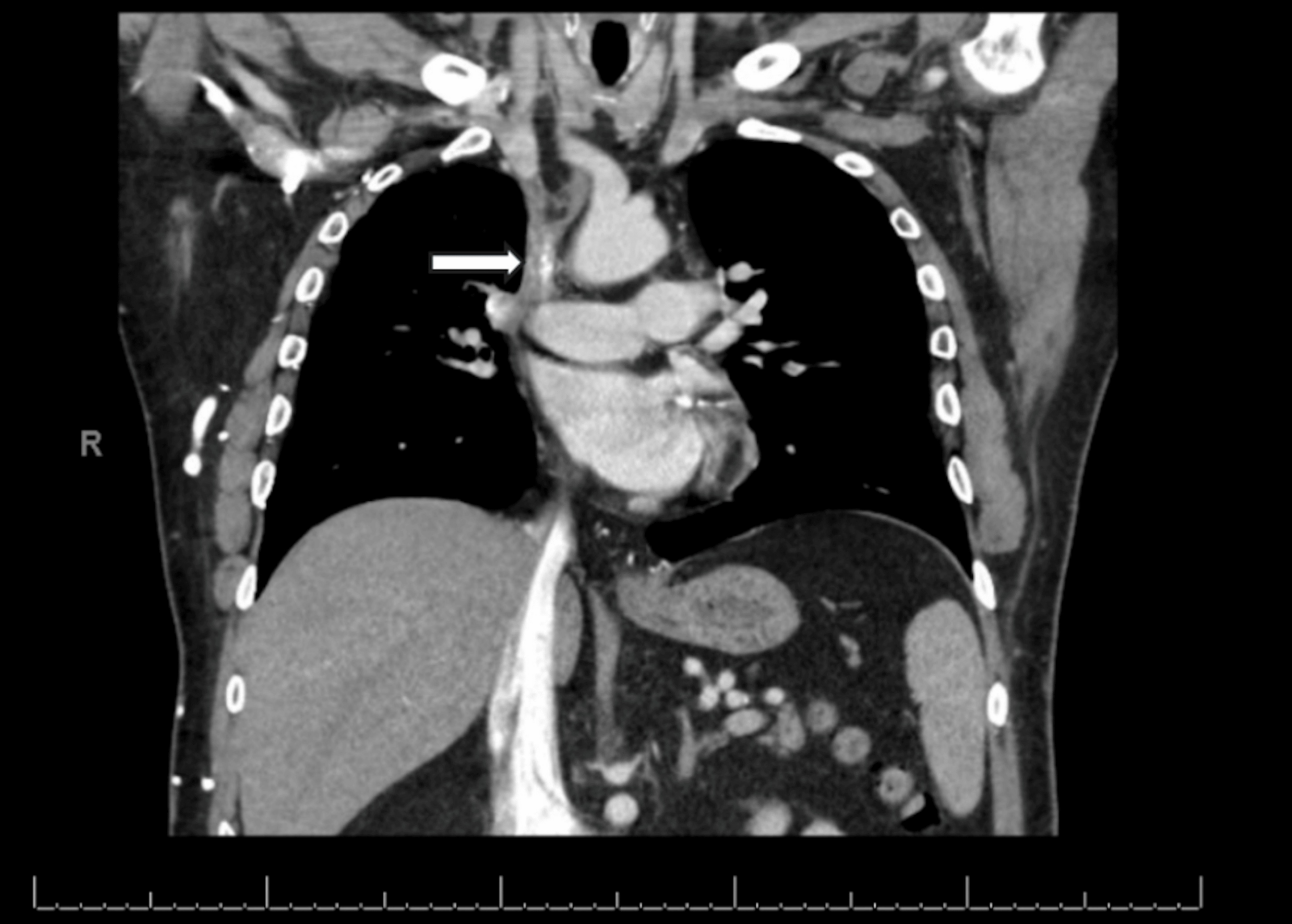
Superior vena cava stenosis and calcification secondary to external pressure from fibrosing mediastinitis. Contrast-enhanced computed tomography of the chest. Coronal view.
